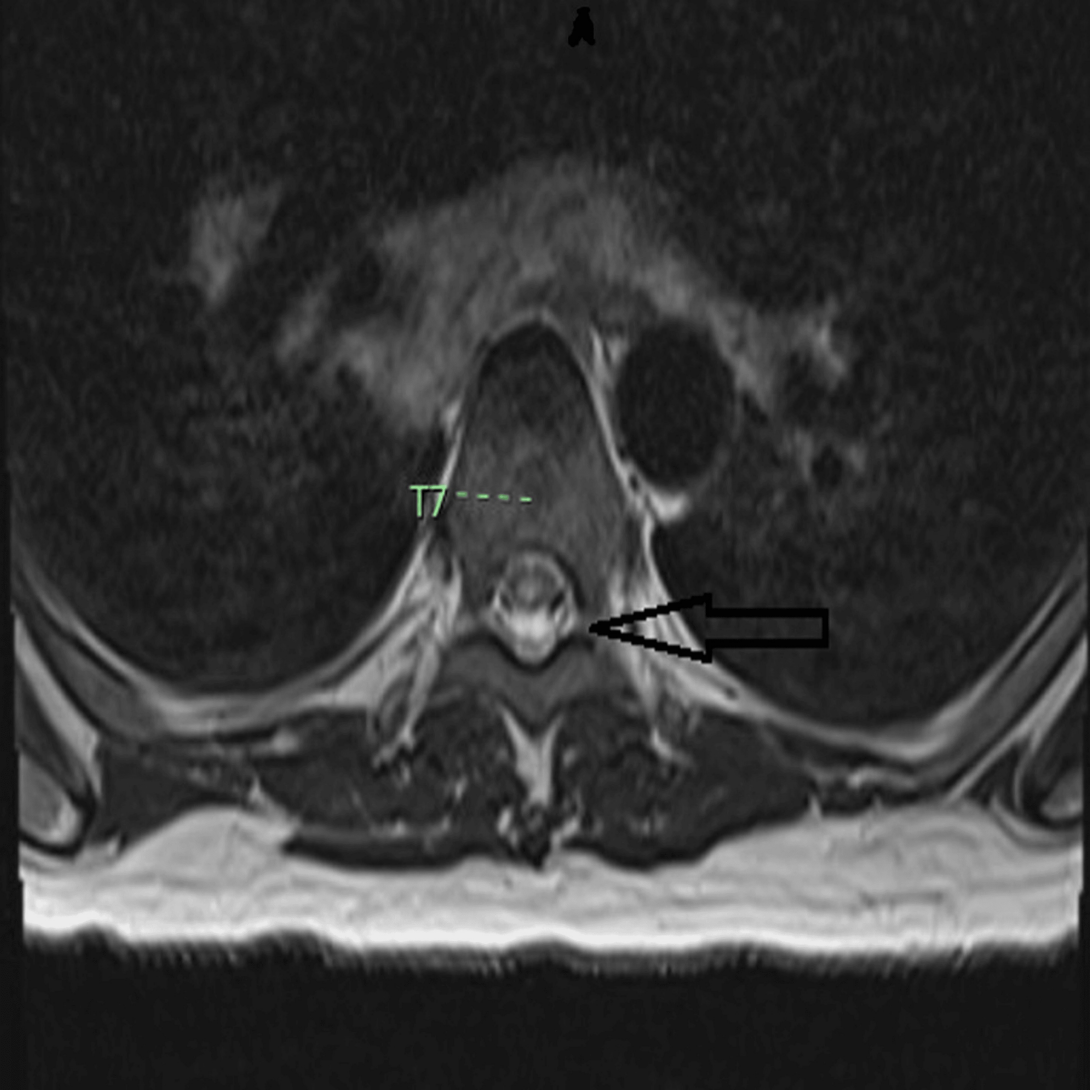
Thoracic spine MRI, T2-weighted image. There is increased signal intensity within the central spinal cord measuring 3 mm x 6 mm at the level of the T7 vertebra.
